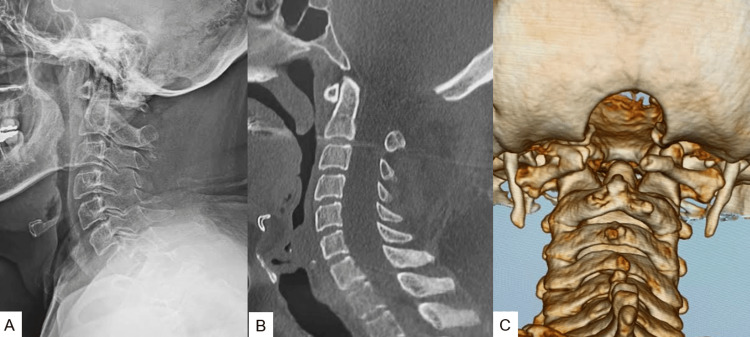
Postoperative images: (A) lateral radiogram, (B) mid-sagittal reconstruction CT, and (C) 3D CT. The foramen magnum was adequately decompressed. CT: computed tomography
